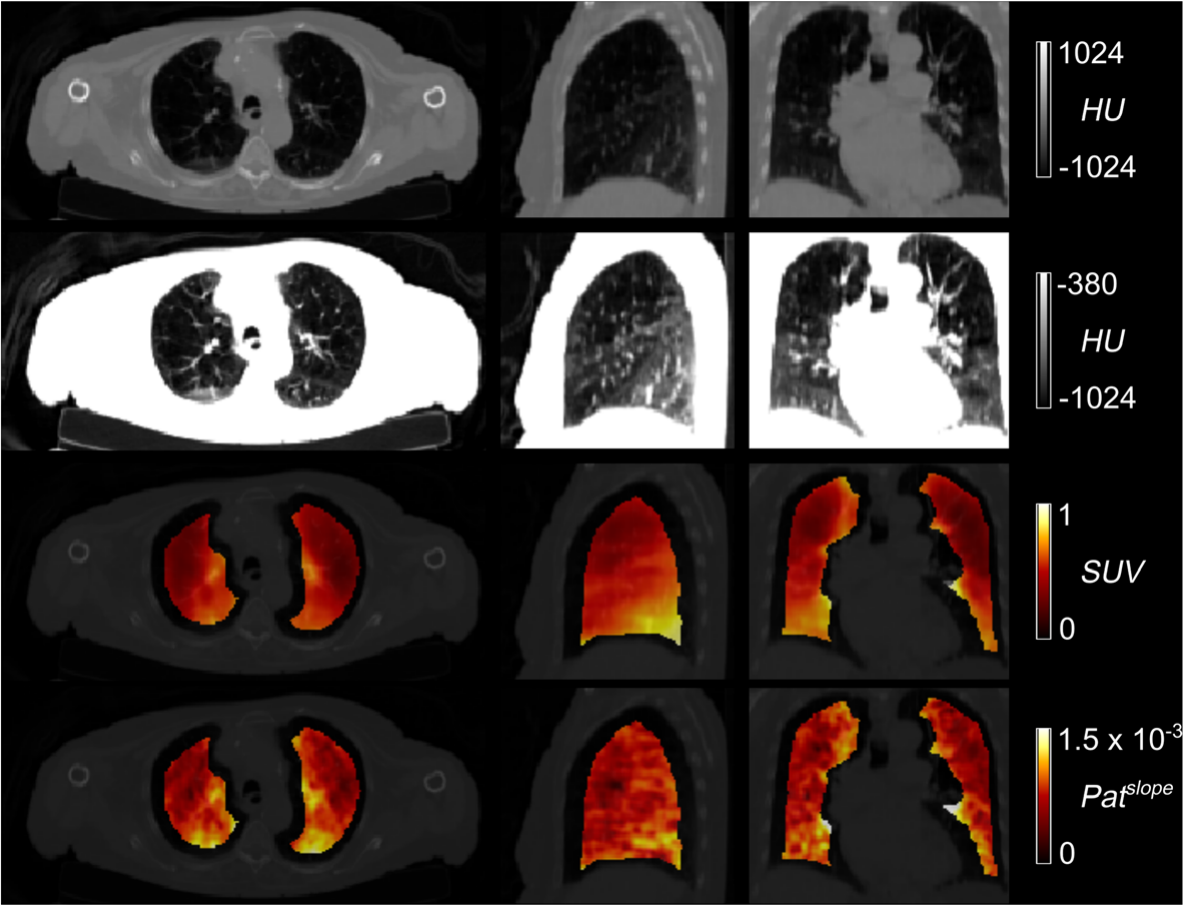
Axial (*left*), coronal (*middle*) and sagittal (*right*) slices for the CT-AC (first and second row) and the averaged (30–60 min) SUV (*third row*) and Pat^slope^ (fourth row) parametric images of a representative COPD patient (M, 66 years). The axial slice was chosen in the upper part of the lung as being representative of tissue loss in COPD. *CT-AC*: computed tomography attenuation correction, *SUV*: standardised uptake value
